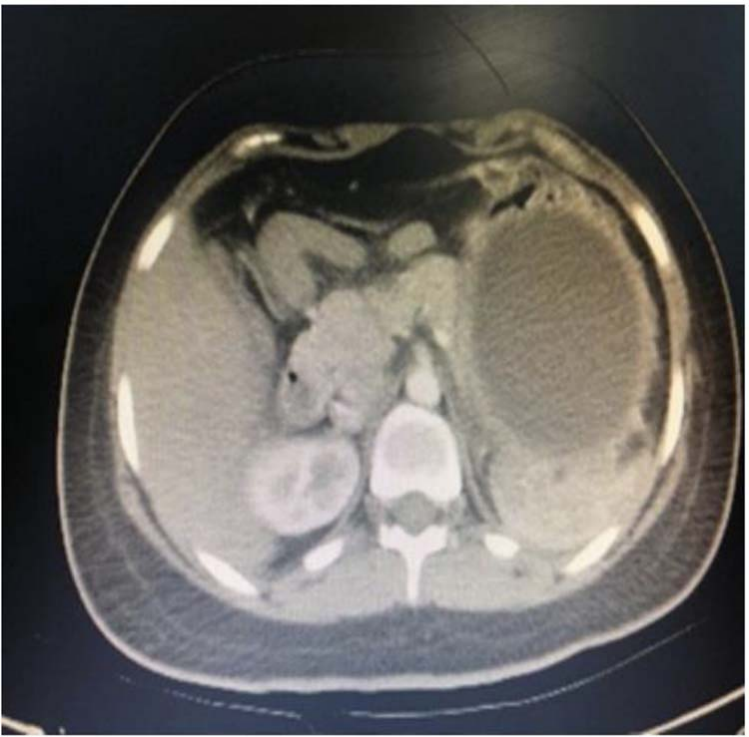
Coronal CT showing the position of the IGB.
